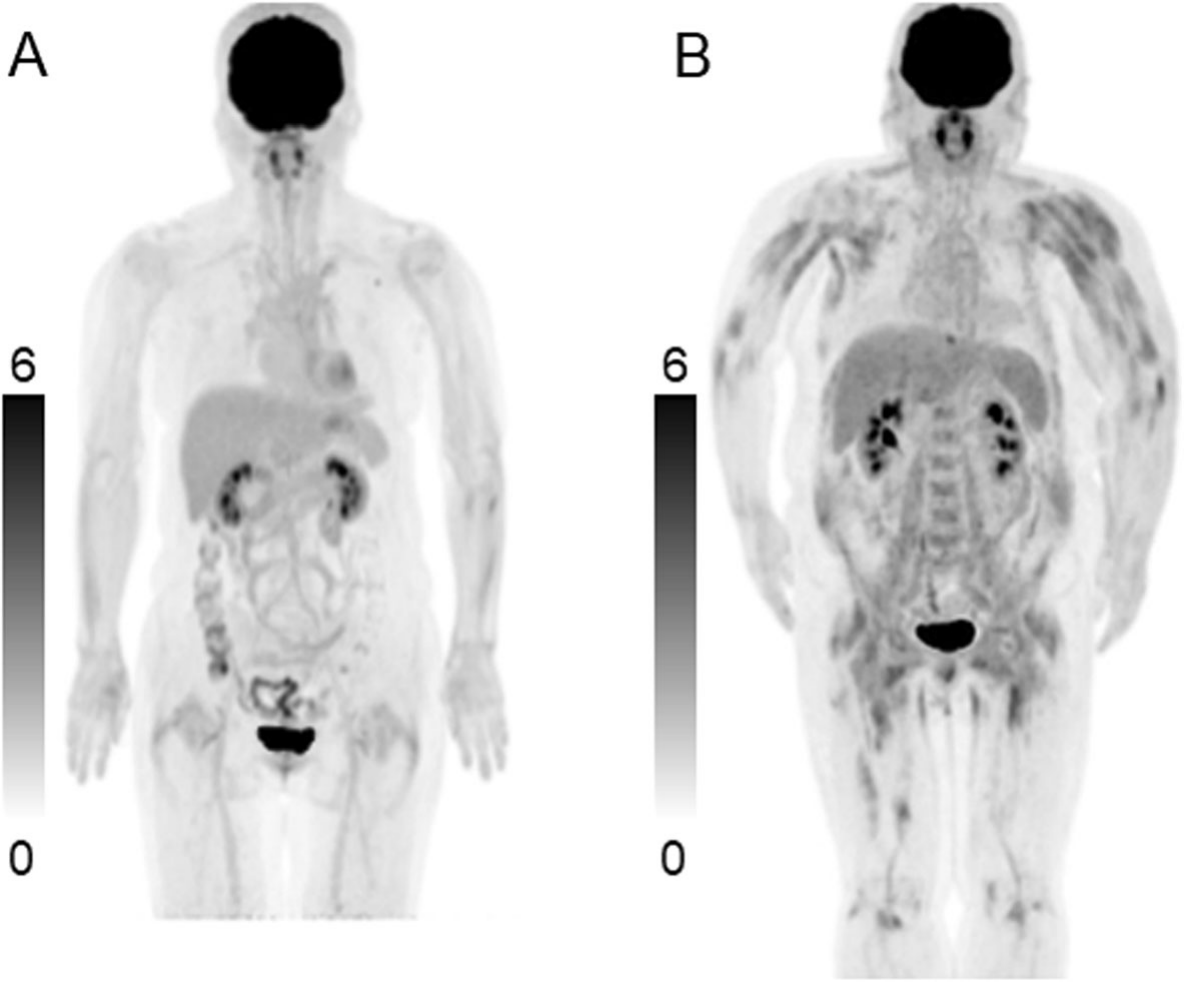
^18^F-FDG PET maximum intensity projection (MIP) image of a 58-year-old female as a control patient shows no increased uptake in the skeletal muscles (a). ^18^F-FDG PET MIP image of a 53-year-old female with dermatomyositis shows diffuse uptake in the proximal skeletal muscles (b).
